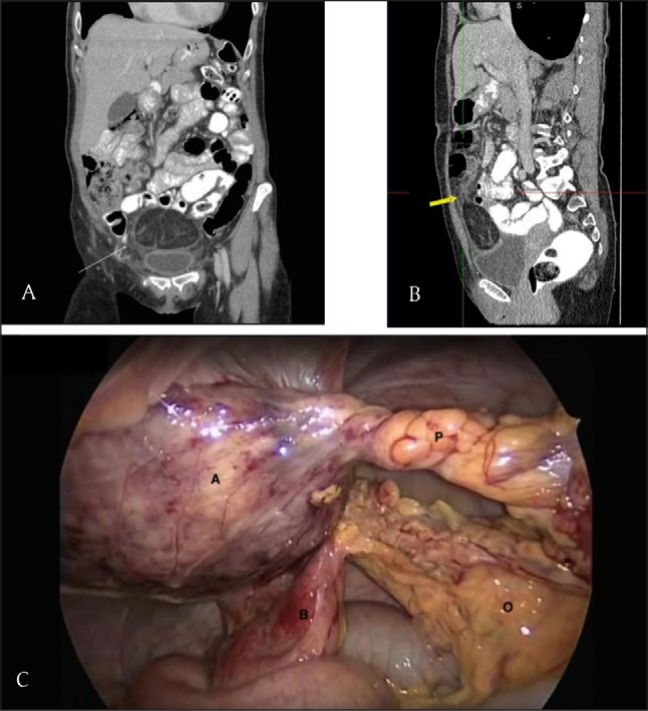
A and B. Coronal and sagittal CT images of an omental-herniated fatty mass (arrows). C. Laparoscopic image of large appendage (A) and its torquated pedicle (P) herniated through the omentum (O). Adhesion of small bowel (B) was present due to inflammation.
